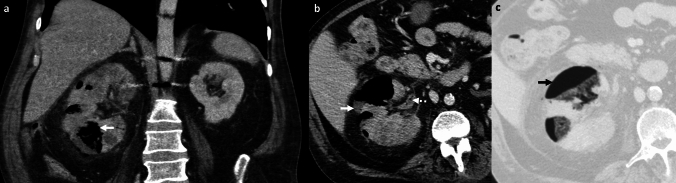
Emphysematous pyelonephritis. A 53-year-old with diabetes with fever, flank pain, and dysuria. CT images show an enlarged right kidney with striated nephrogram and air foci (white arrow, a). There is an extrarenal extension with an air-fluid level (white arrow, b). Air also extends to the renal pelvis with urothelial enhancement (dotted white arrow, b). Lung window images show emphysematous changes and air-fluid levels (black arrow, c). Findings are consistent with Class 3A emphysematous pyelonephritis (with perinephric extension). The patient was treated with antibiotics and percutaneous drainage
